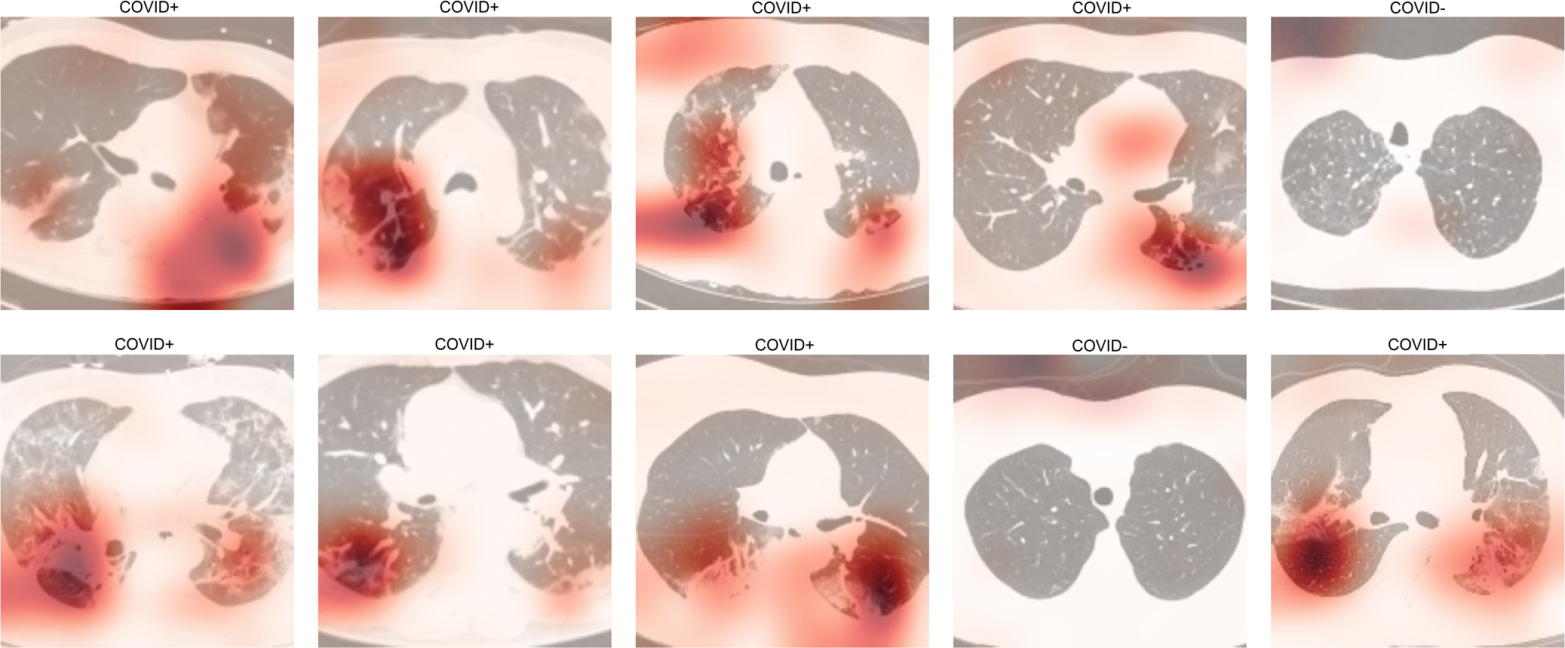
Grad-CAM over CT scans of COVID19+ and COVID19- pneumonia patients. On top right, DCD correctly diagnosed the scan of a COVID19- patient with PNA who showed signs of ground glass opacity.
